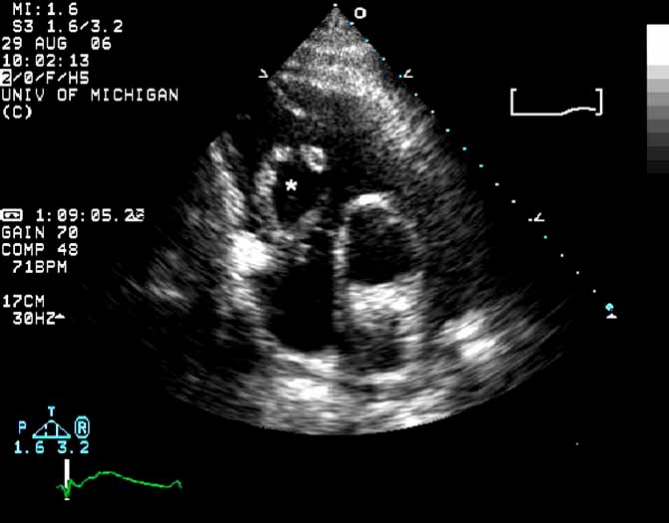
Pulmonary embolism in transit. This short axis tranthoracic echocardiogram image shows an embolism (^*^asterix) passing through the tricuspid valve into the right ventricle.
